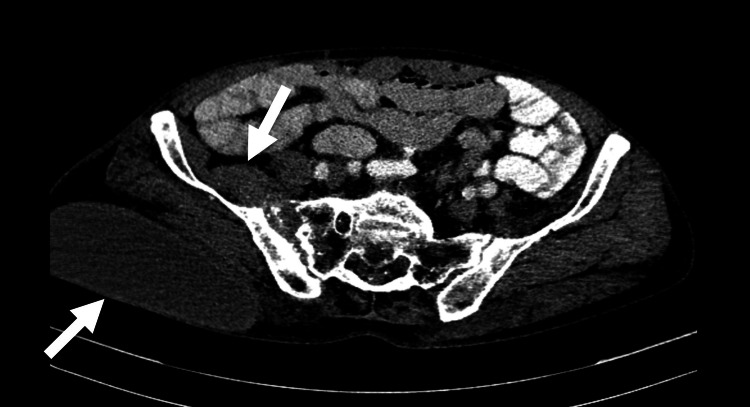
Abdominopelvic axial CT with oral and intravenous contrast CT abdomen demonstrates a hypodense collection arising from the distal portion of the iliopsoas muscle, with extension through a probable fistula to soft tissue of the right gluteal region.
